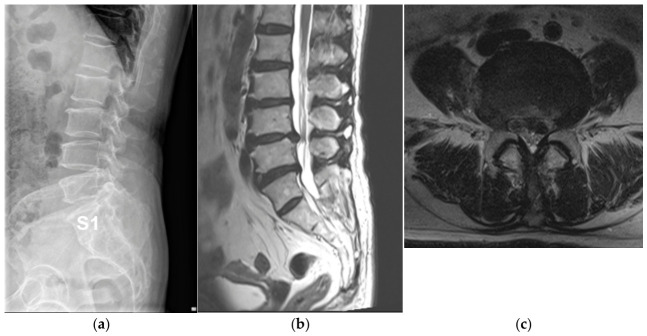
Representative concordant case in a patient with partial lumbarization. A 62-year-old female patient demonstrated partial lumbarization of S1. (a) Lumbar spine radiographs show transitional anatomy consistent with partial lumbarization. (b) Sagittal lumbar MRI demonstrates disc herniation at the L4–5 level. (c) Axial MRI shows left L5 nerve root compression. Clinically, the patient presented with pain without motor weakness. Electrophysiological evaluation demonstrated abnormal findings in the tibialis anterior and peroneus longus muscles, consistent with L5 radiculopathy. These findings demonstrate concordance between MRI-defined anatomical compression and EMG-identified functional nerve root involvement.
